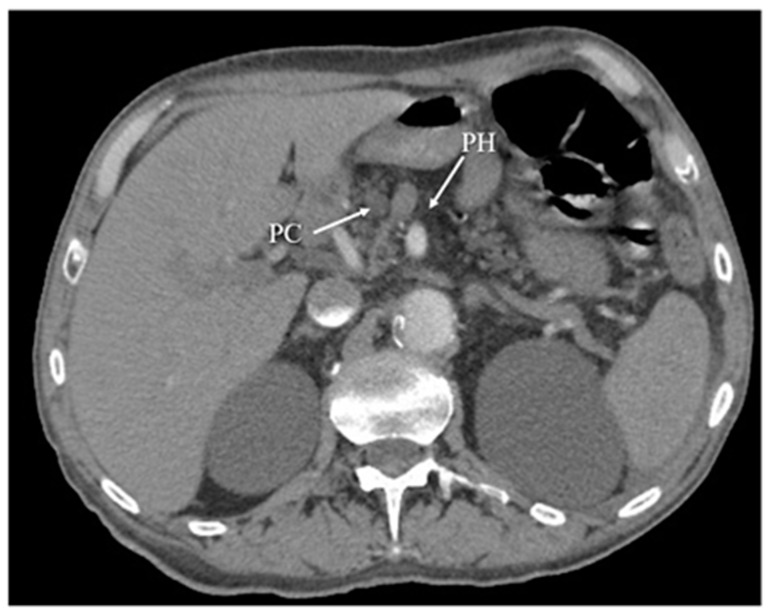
Chronic pancreatitis, with atrophy of pancreatic head (PH) parenchyma and pseudocyst (PC) in this region. The arterial phase of CT.
